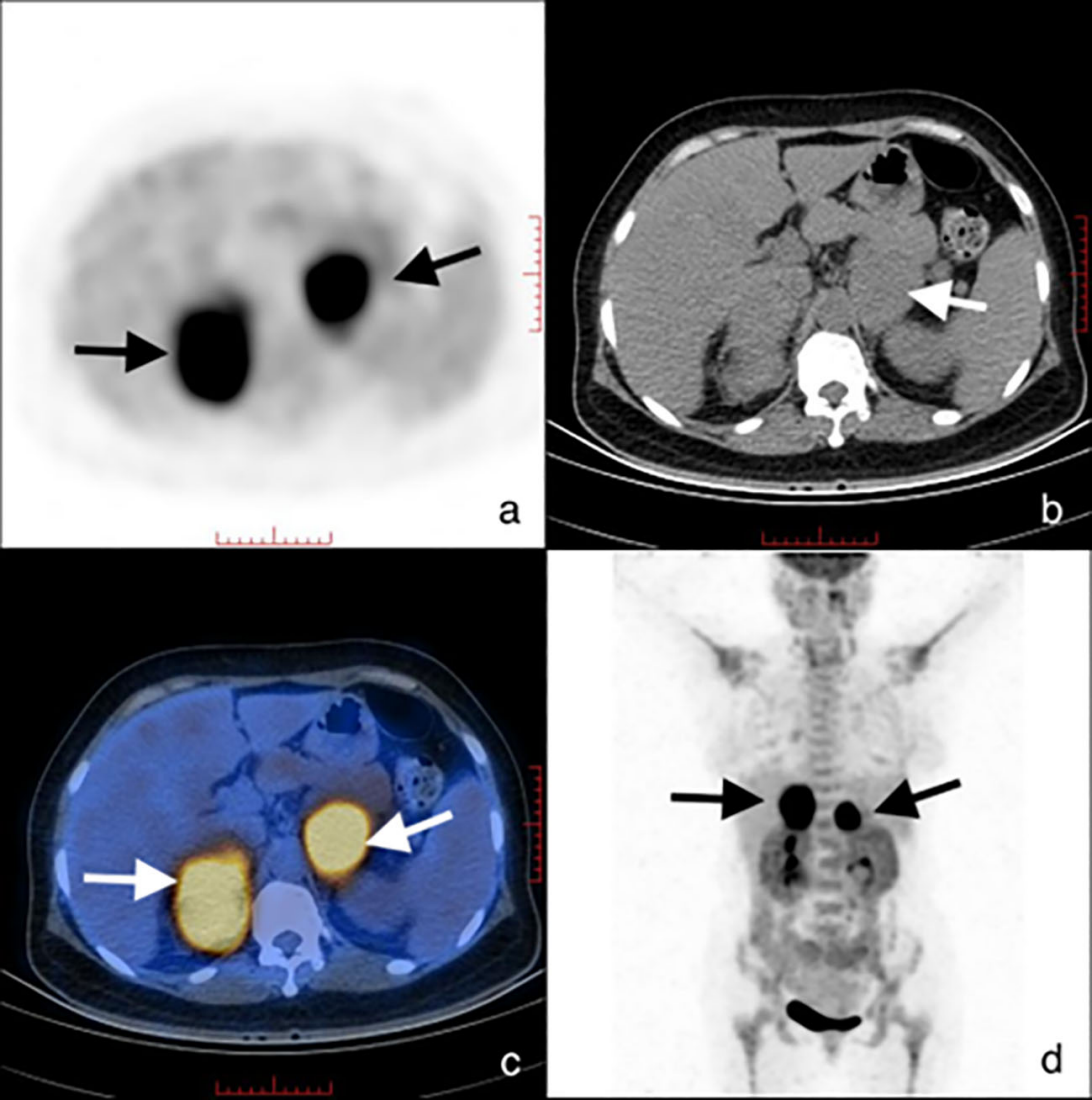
^18^F-FDG PET image (a), transaxial CT (b), PET-CT image (c), and torso PET image (d) revealed uptake in bilateral adrenal glands with SUV_max_ of 17.9 (noted by arrows). SUV, standard uptake value.
